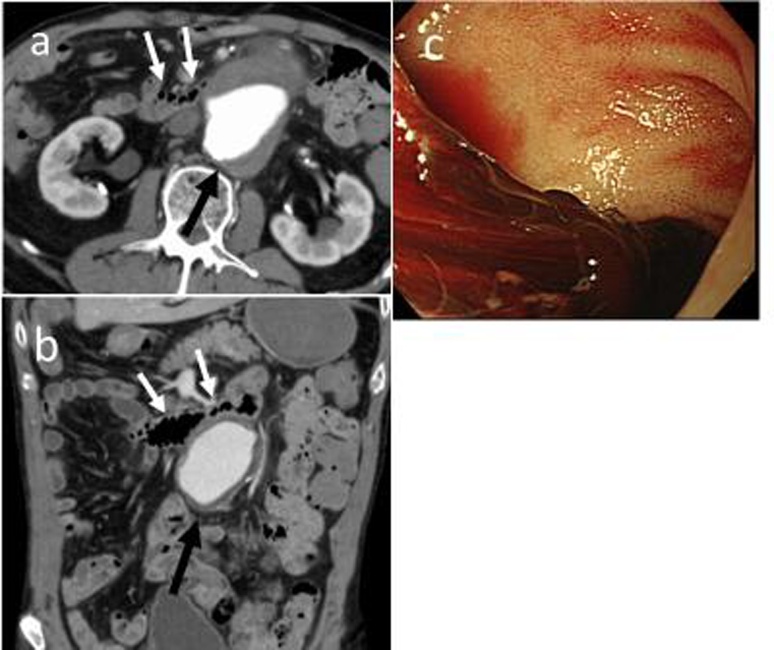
Abdominal computed tomography (CT) scan and upper gastrointestinal endoscopy (UGE). Axial (a) and coronal (b) abdominal CT scan revealed that the abdominal aortic aneurysm (black arrow) below the renal artery was dilated 7.6 × 5.4 cm, which compressed the duodenum (white arrows). (c) UGE revealed a large amount of fresh blood and blood clots in the bulb of the duodenum.
